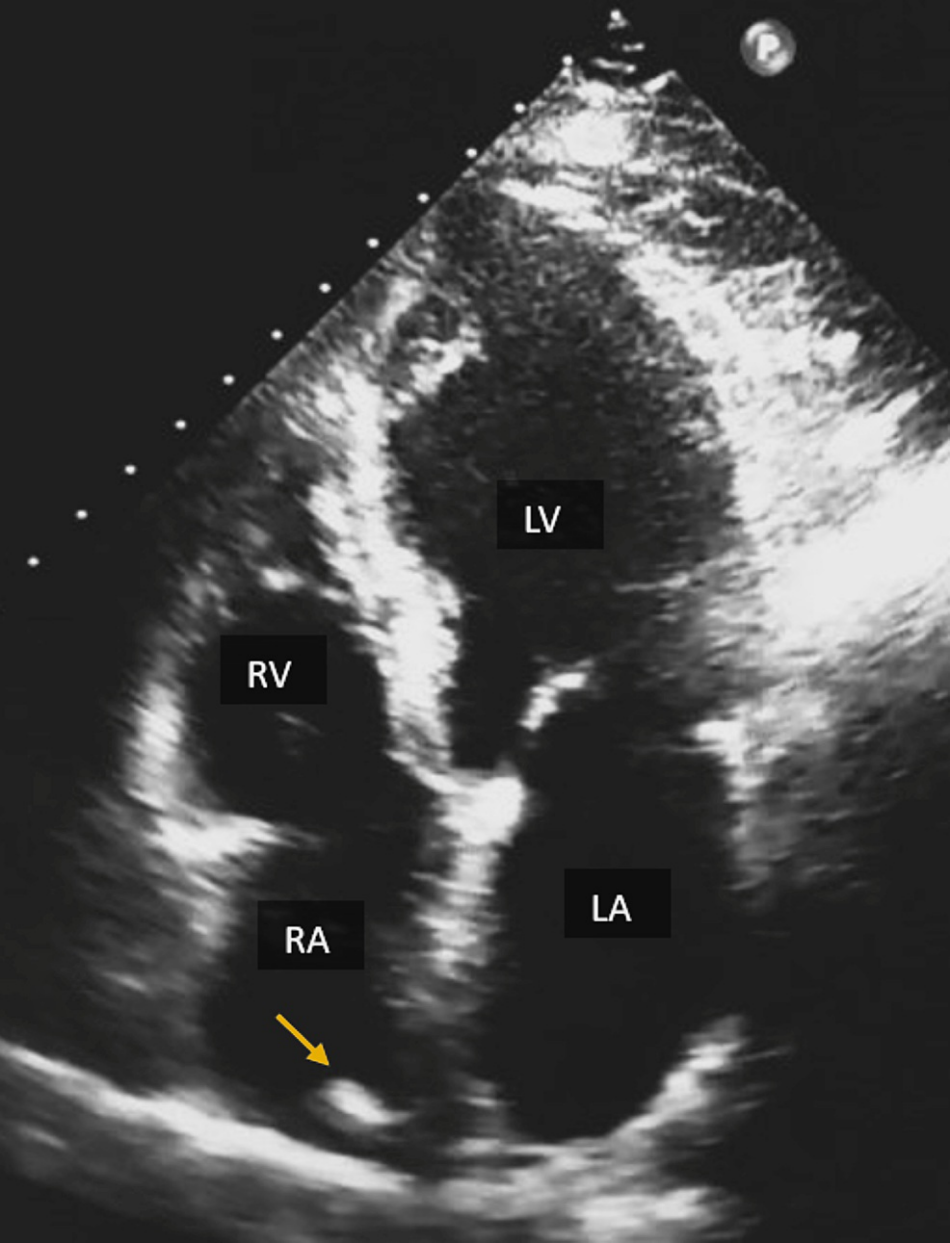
The apical four-chamber view visualizes mildly dilated left (LV) and right ventricles (RV) with a ventricular septal bulge and mild dilatation of the left atrium (LA). Transthoracic echocardiography also reveals the presence of the crista terminalis, which is a normal variant in the right atrium (RA) (arrow).
